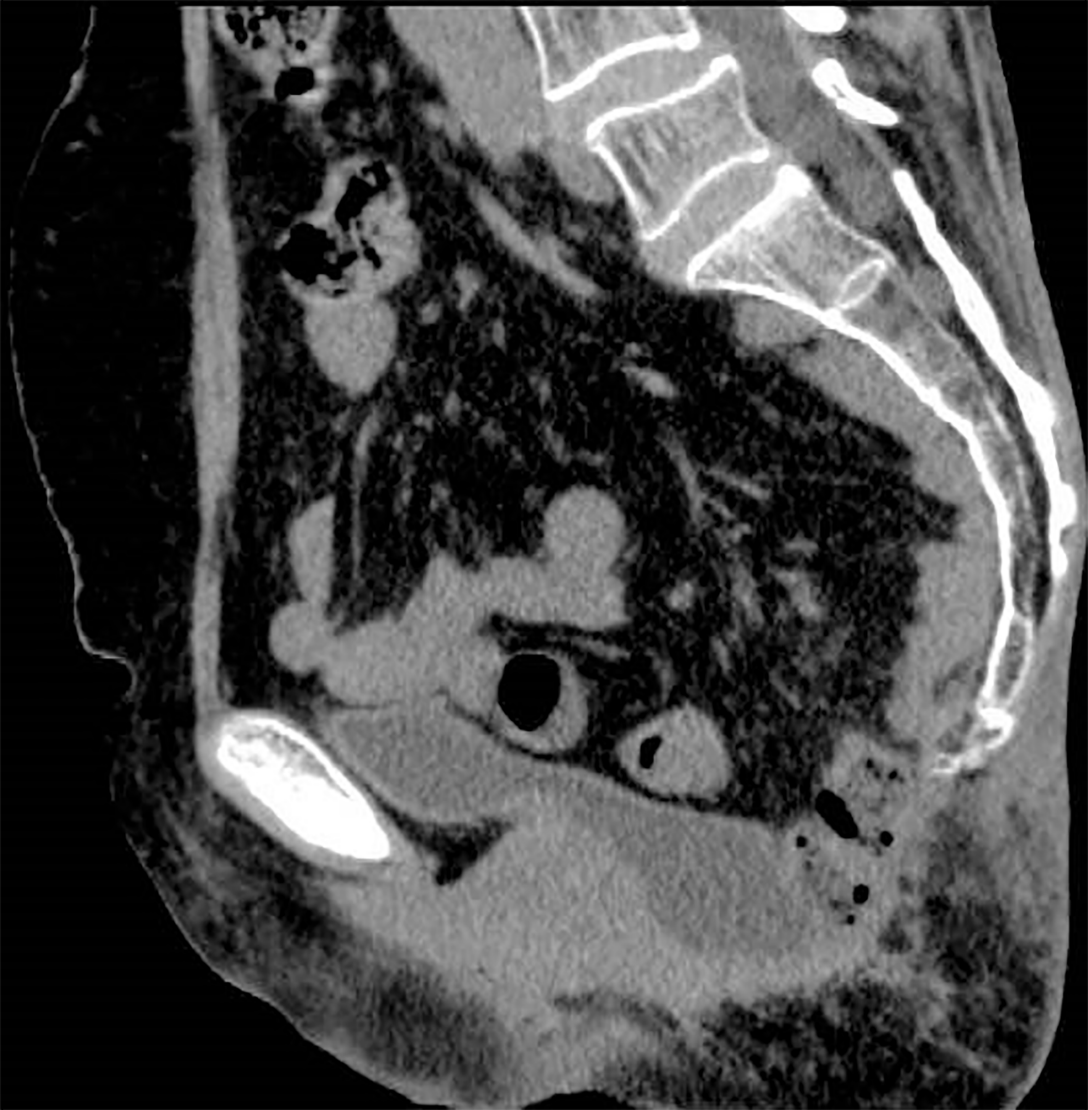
CT view of the hernia postoperatively.
